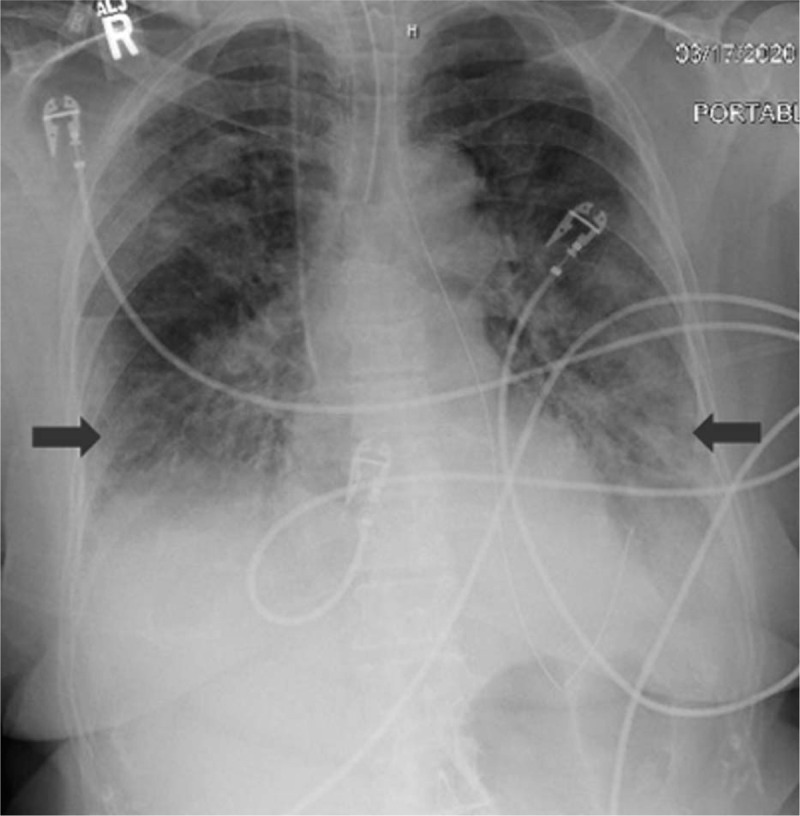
Chest X-ray showing bilateral infiltrates worsened in the lower lung fields.
